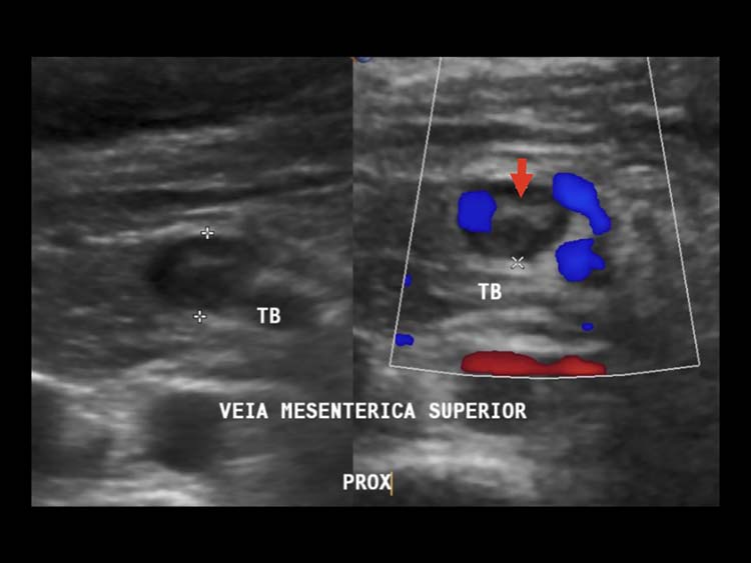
Doppler ultrasound showing superior MVT. Arrow: mesenteric vein thrombus.
